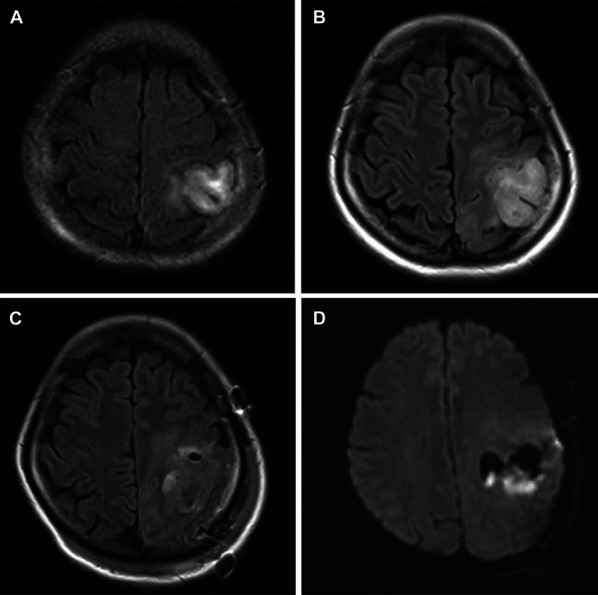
MRI of a 37-year-old woman showing a left parietal brain tumor. A, She was asymptomatic and incidentally diagnosed. The patient was followed up in an outpatient clinic for 11 years; B, the tumor shows gradual expansion. We performed awake craniotomy. C, Image showing almost 90% tumor removal. Nineteen hours after surgery, sensory disturbances in her right hand, dysarthria, and dysgraphia appeared. D, Diffusion-weighted MRI showing cerebral ischemia in the deep white matter.
